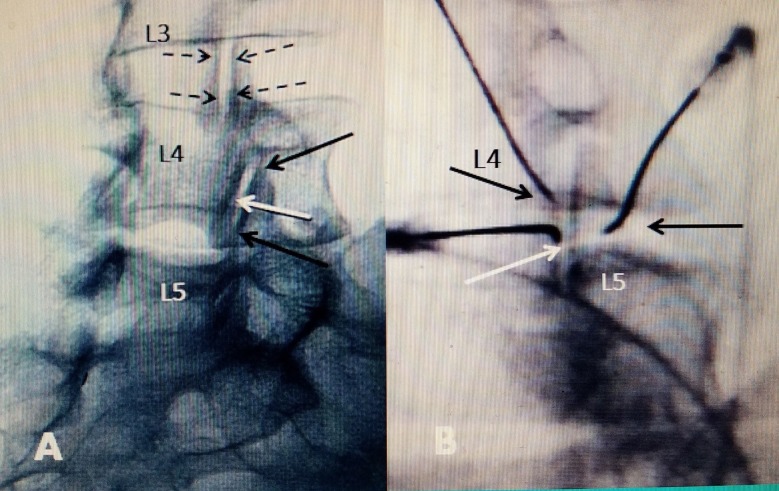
Positioning of bipolar RF electrodes Electrodes positioned within 10 mm of each other for bipolar effect A: At L3-4, dotted black arrows represent the positioning of electrodes on either side of the facet joint capsule. The capsule covers the posterior joint and radiofrequency (RF) current heats across the posterior capsule. At the L4-5 facet joint, the two bipolar electrodes represented by solid black arrows are positioned lengthwise along the superior and the inferior part of the joint space, creating a bipolar RF effect along the length of joint. One electrode is on the edge of the inferior facet L4 and the other on the superior facet of L5 along the L4-5 joint space. The solid white arrow represents an intrafacet electrode positioning within the joint space. B: Oblique radiograph during the actual procedure covering the L4-5 fact joint. Black arrows on two RF electrodes on either side of the joint space are the two bipolar electrodes across the L4-5 joint space. The white arrow is an RF electrode with a curved tip positioned within the joint space.
